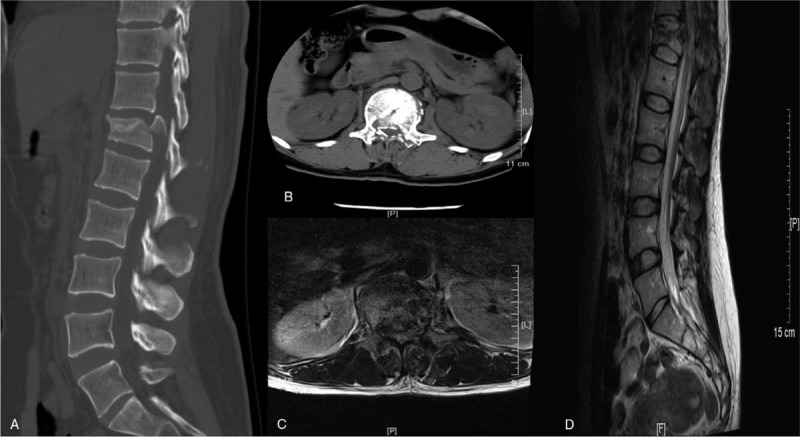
Imaging of the patient on admission. On the sagittal (A) and axial (B) computed tomography, the retropulsion of bone fragments into the spinal canal. Magnetic resonance imaging of T2-weighted image (C) and (D) also showed that bone fragments had retropulsed into the spinal canal and dural sac was severely compressed.
